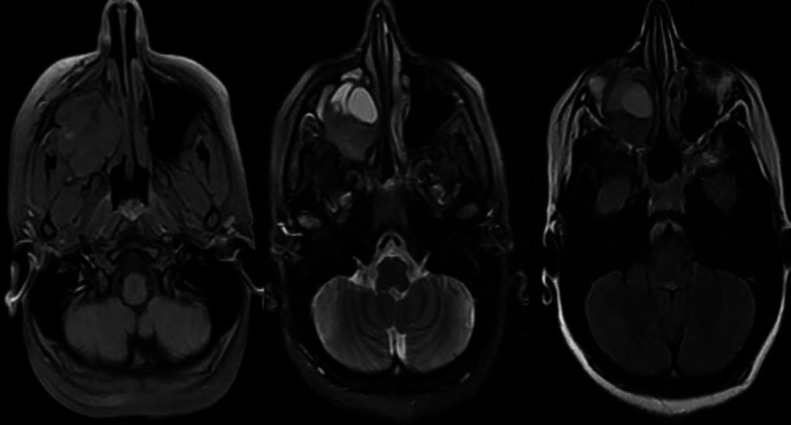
Axial T1, T2, and T2 fluid-attenuated inversion recovery weighted magnetic resonance imaging. There is evidence of abnormal enlarge well-marginated border, and heterogeneous signal soft tissue mass was observed in all sequences
